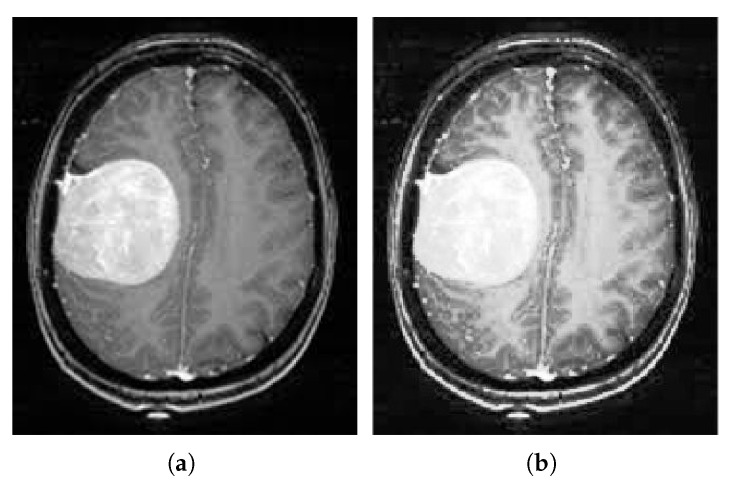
Linear contrast stretch outcomes. (a) Input brain MRI, (b) Final contrast stretch image.
